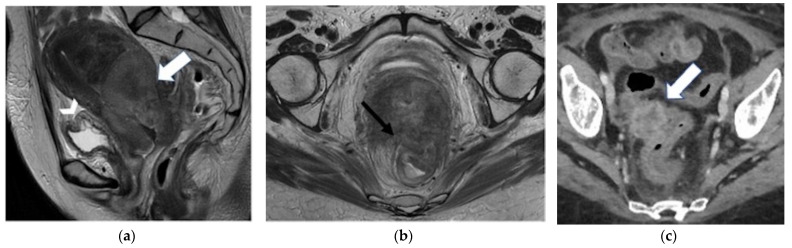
(a,b) A 46-year-old patient who underwent chemoradiation therapy for recent cervical cancer. (a) Sagittal and (b) axial FSE T2-weighted images show a soft-tissue mass in the cervix, extended posteriorly to the rectal wall (white arrow), causing luminal narrowing (black arrow). Note the bullous oedema of the posterior bladder wall mucosa (arrowhead). (c) A 58-year-old patient who underwent radical hysterectomy with BSO and chemoradiation therapy for cervical cancer. Axial post-contrast CT image shows a nodular mass in the right pelvis infiltrating the distal sigma (arrow).
